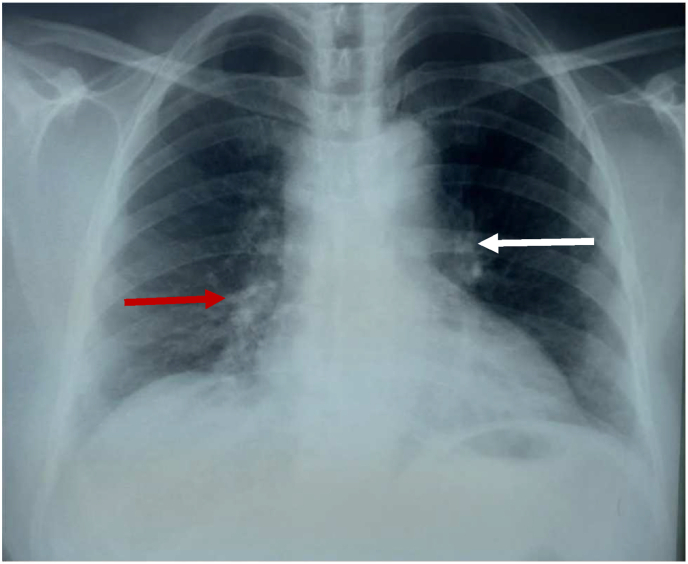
Chest X-ray: Left hilar opacity (white arrow) and a right paracardiac opacity (red arrow). (For interpretation of the references to colour in this figure legend, the reader is referred to the Web version of this article.)
